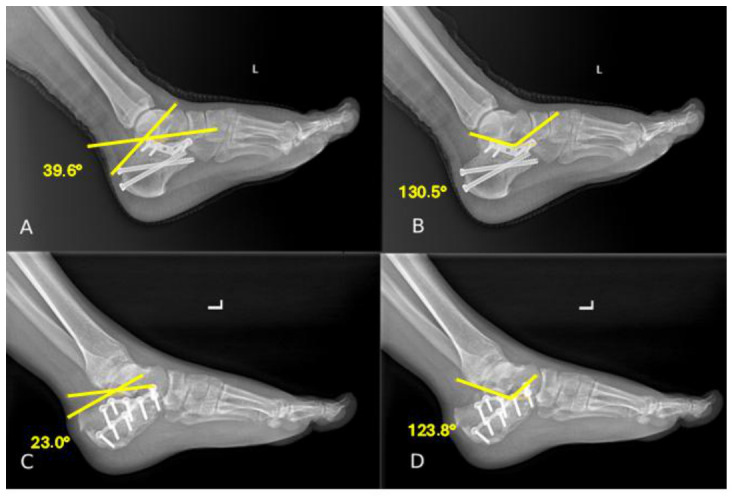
Postoperative lateral foot radiographs demonstrating Böhler and Gissane angle measurements. The yellow lines indicate the reference lines used for Böhler and Gissane angle measurements. (A) Postoperative Böhler angle measurement in a patient treated with the sinus tarsi approach. (B) Postoperative Gissane angle measurement in the same patient. (C) Postoperative Böhler angle measurement in a patient treated with the extensile lateral approach. (D) Postoperative Gissane angle measurement in the same patient.
